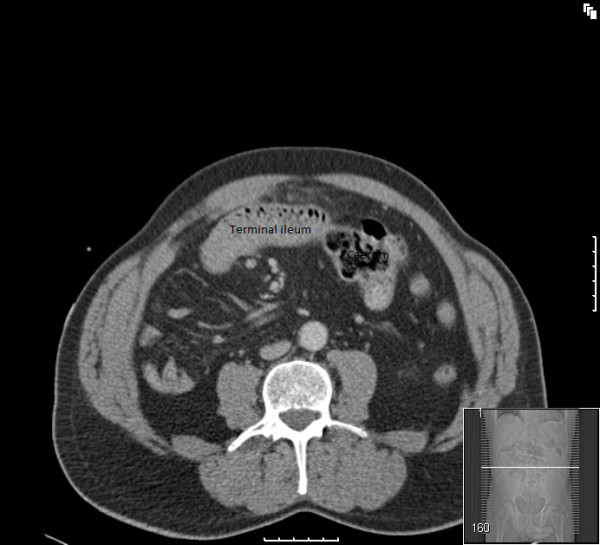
CT scan showing caecum on the left side of the abdomen and terminal ileum entering the caecum from the right side.
